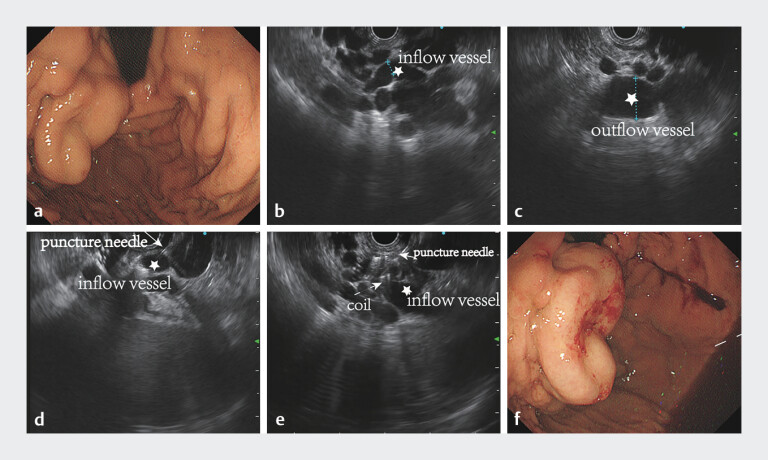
Endoscopy and endosonography images.
